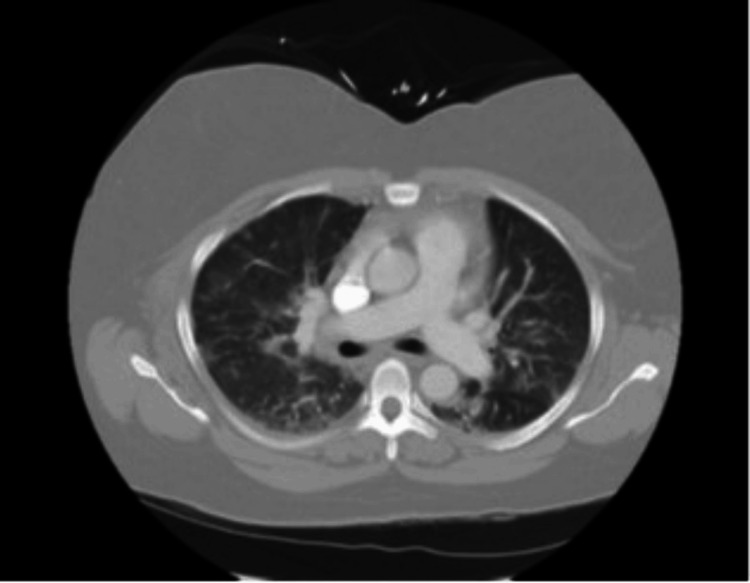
A CT chest with and without contrast conducted upon admission revealed the presence of lung infiltrates
